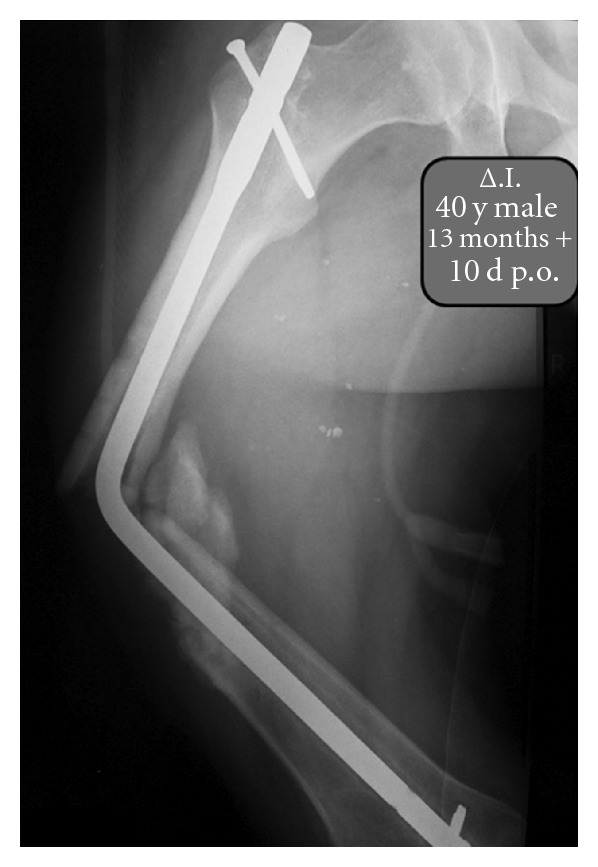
Radiographic image showing refracture of the femoral shaft and a bending deformation of the intramedullary nail.
